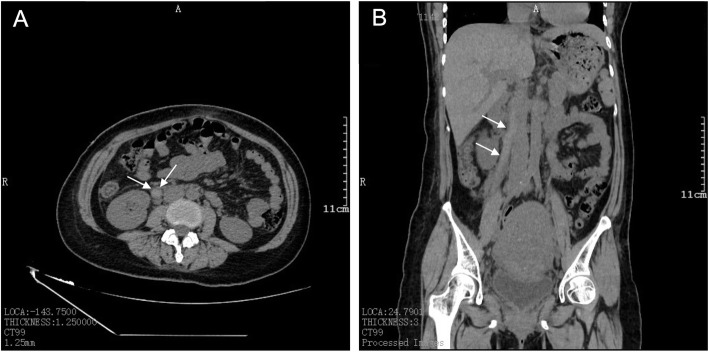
CT image showing right ovarian vein thrombosis. A Superior-inferior view. B Anterior-posterior view
